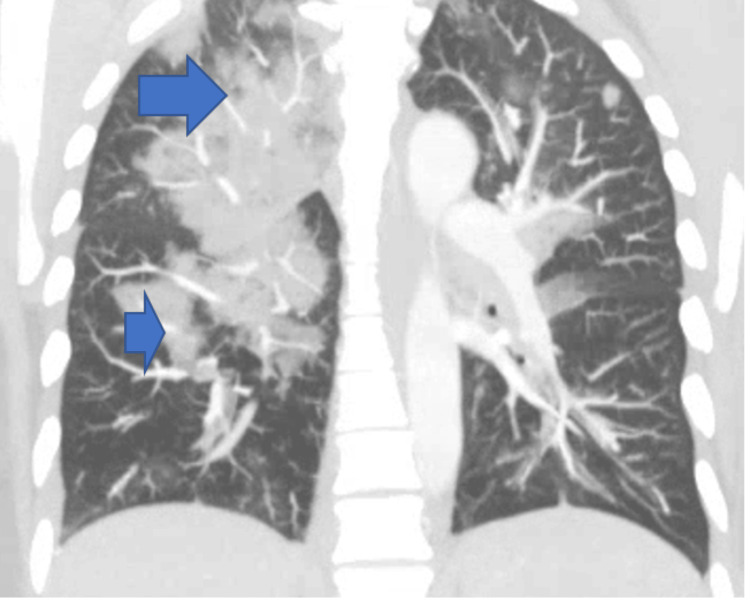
Computed tomography of the chest (sagittal section) showing bilateral airspace disease (bold blue arrows on right-sided findings).
